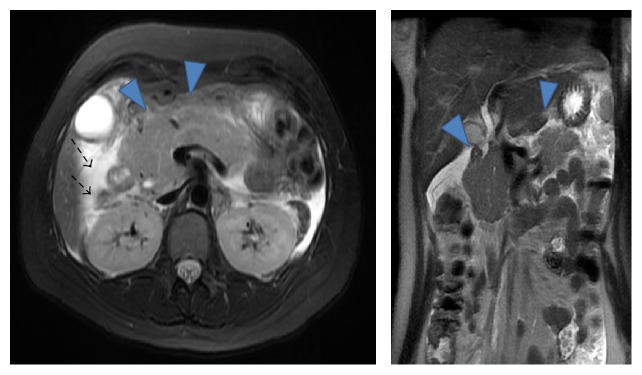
MRI T2W. (▼) Enlargement of the pancreatic body. (→) Inflammation of acute pancreatitis, extending to fat tissue and reaching beyond the lower pole of the kidney.
